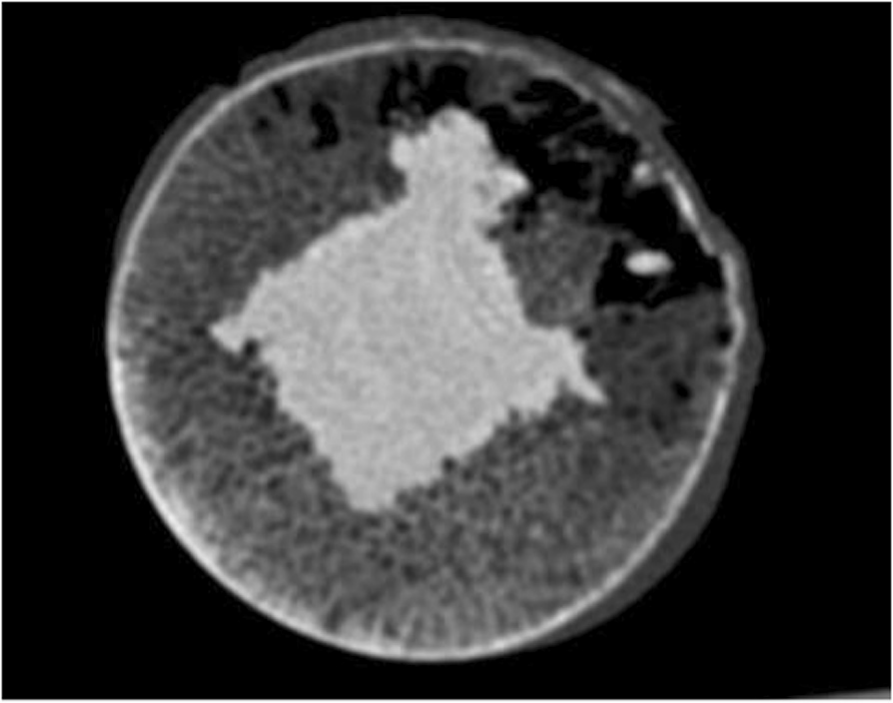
CT scan of the pure cemented cubic centimetre.
